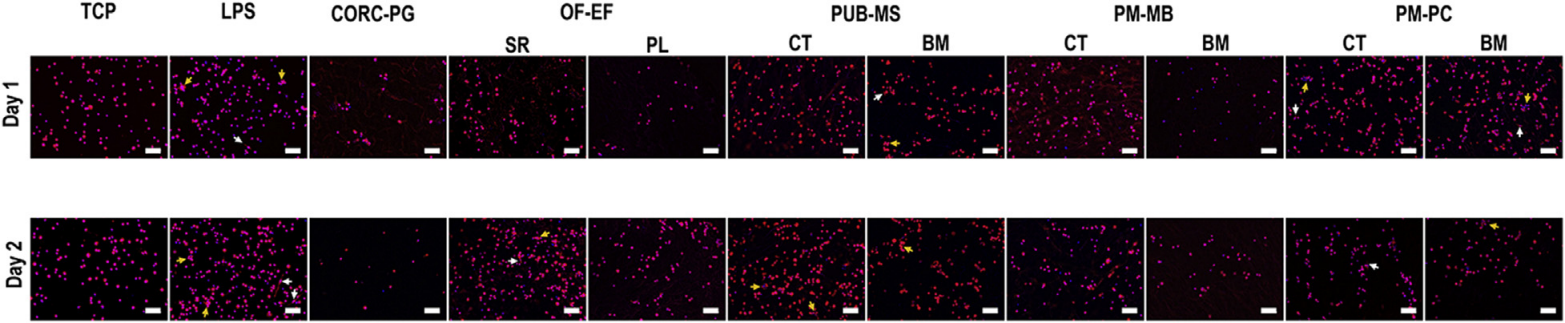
Immunocytochemistry (red: cytoskeleton; blue: nuclei) analysis of THP-1 revealed the presence of elongated cells (white arrows; LPS at days 1 and 2, SR side of OF-EF at day 2, BM side of PUB-MS at day 1, CT side of PM-PC at days 1 and 2, and BM side of PM-PC at day 1) and cell clusters (yellow arrows; LPS at days 1 and 2, SR side of OF-EF at day 2, BM side of PUB-MS at day 1 and both sides at day 2, both sides of PM-PC at day 1, and BM side of PM-PC at day 2). Scale bars 100 μm.
